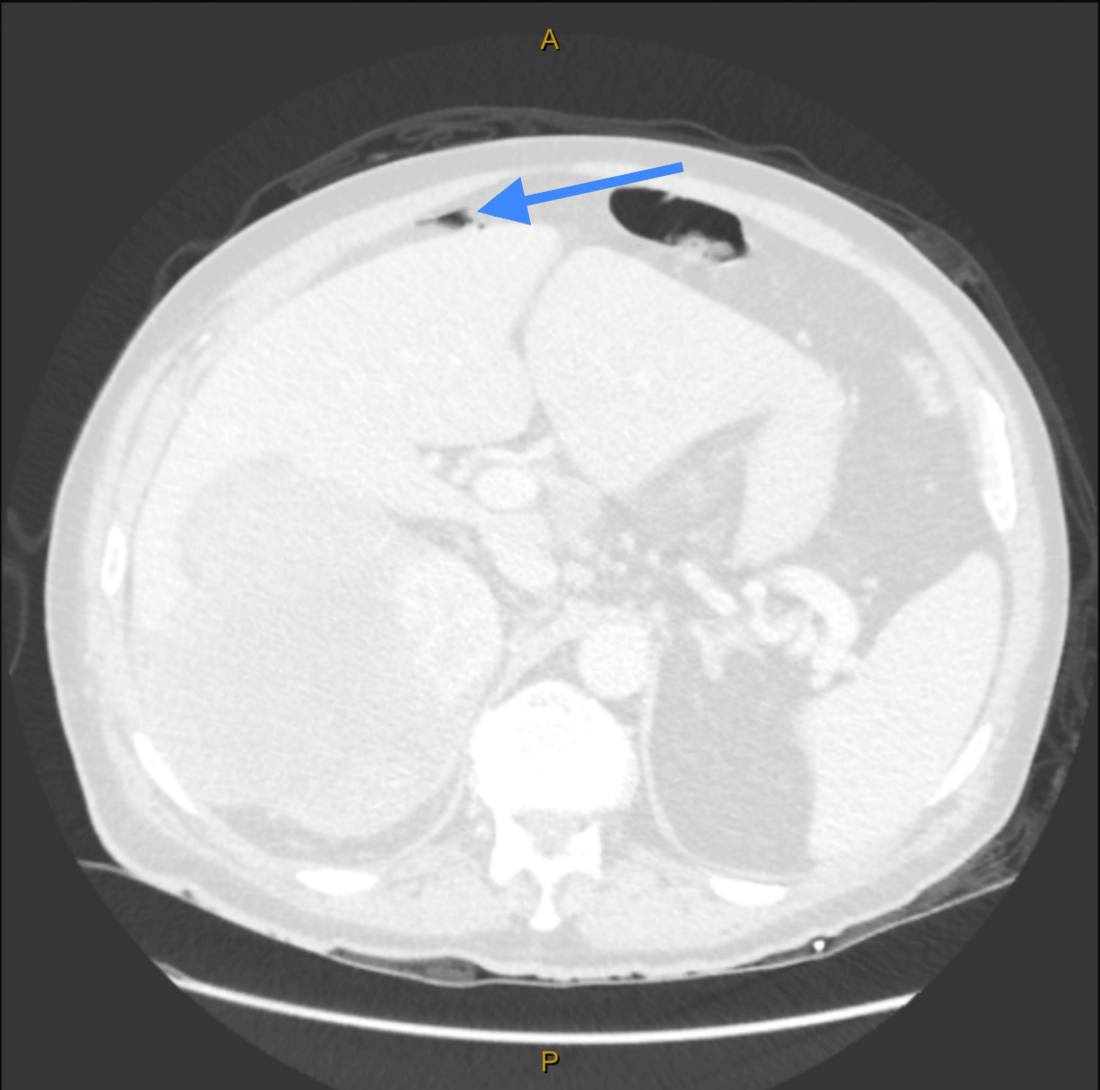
CT abdomen and pelvis with intravenous contrast demonstrating bowel perforation with pneumoperitoneum (arrow).
